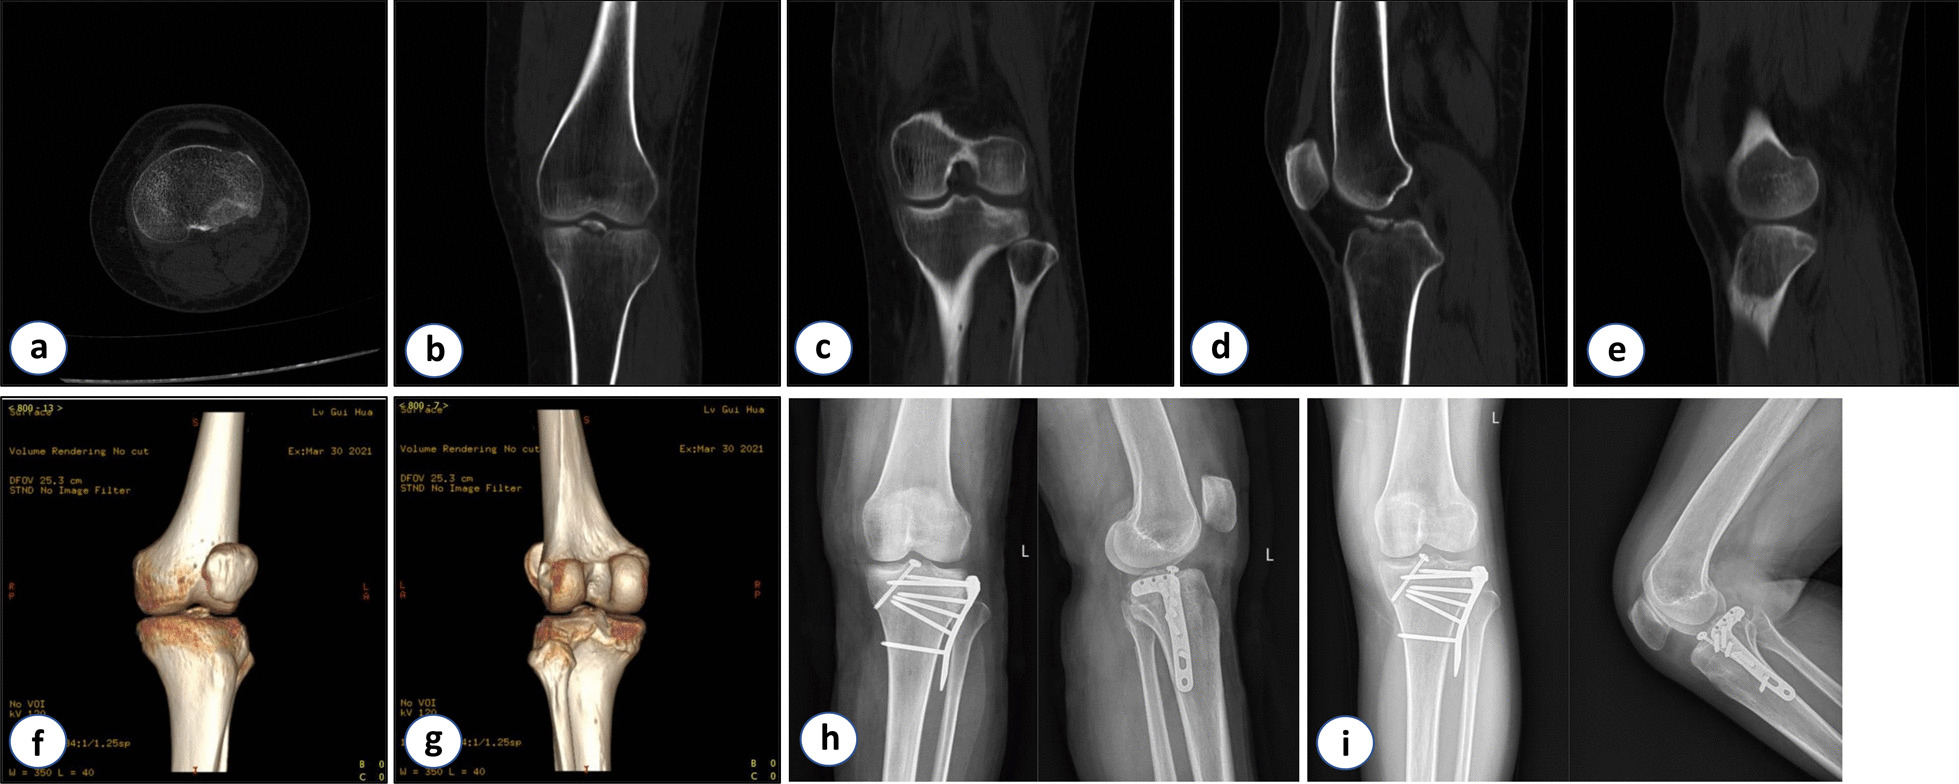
A patient with type III flexion-valgus tibial plateau fracture. a–e Preoperative transverse, coronal and sagittal CT images of the tibial plateau. f, g 3D reconstruction images of the tibial plateau. h Postoperative X-ray examination of the knee joint (3 days after surgery). i Postoperative X-ray examination of the knee joint (8 weeks after surgery)
